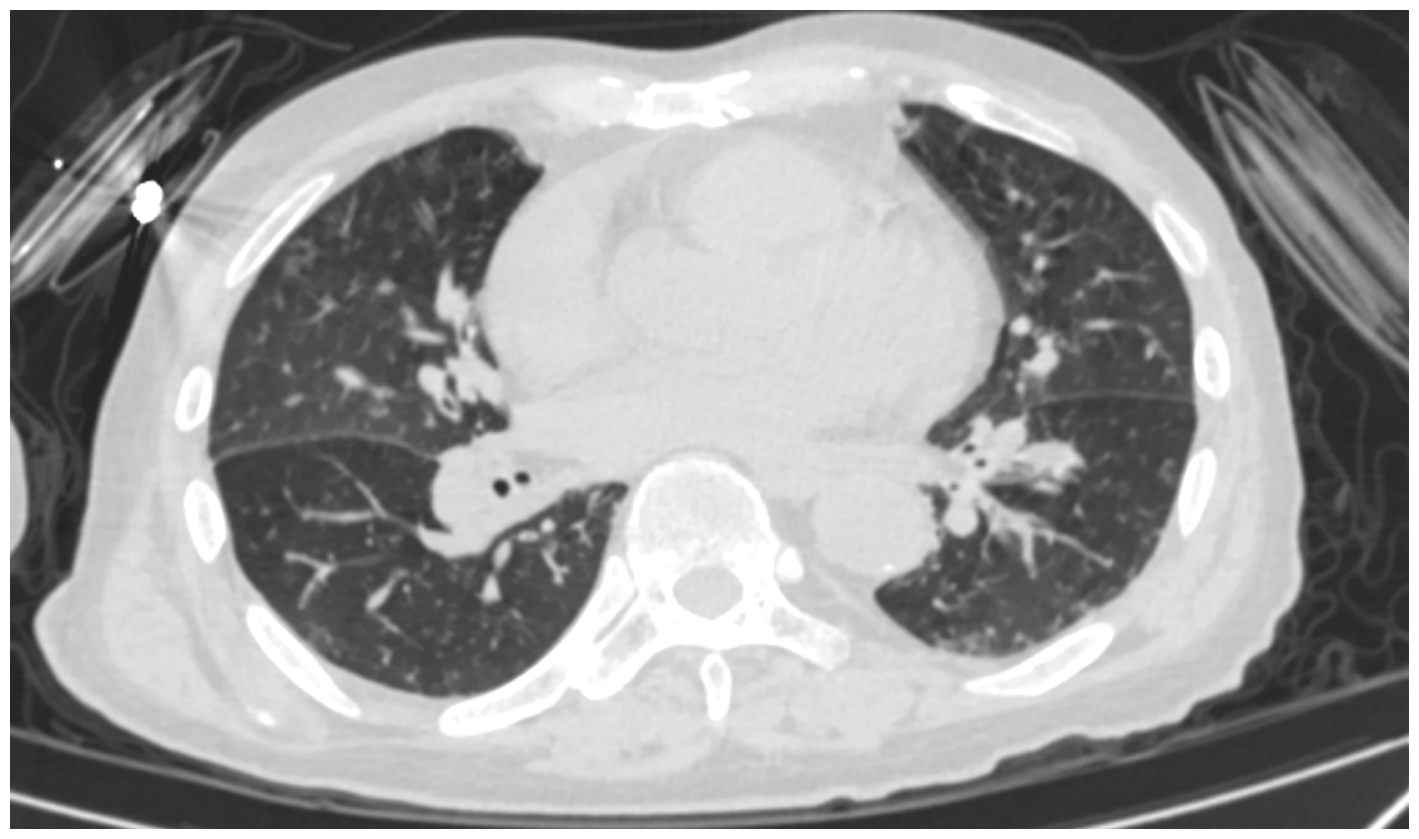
Chest CT of the patient.
